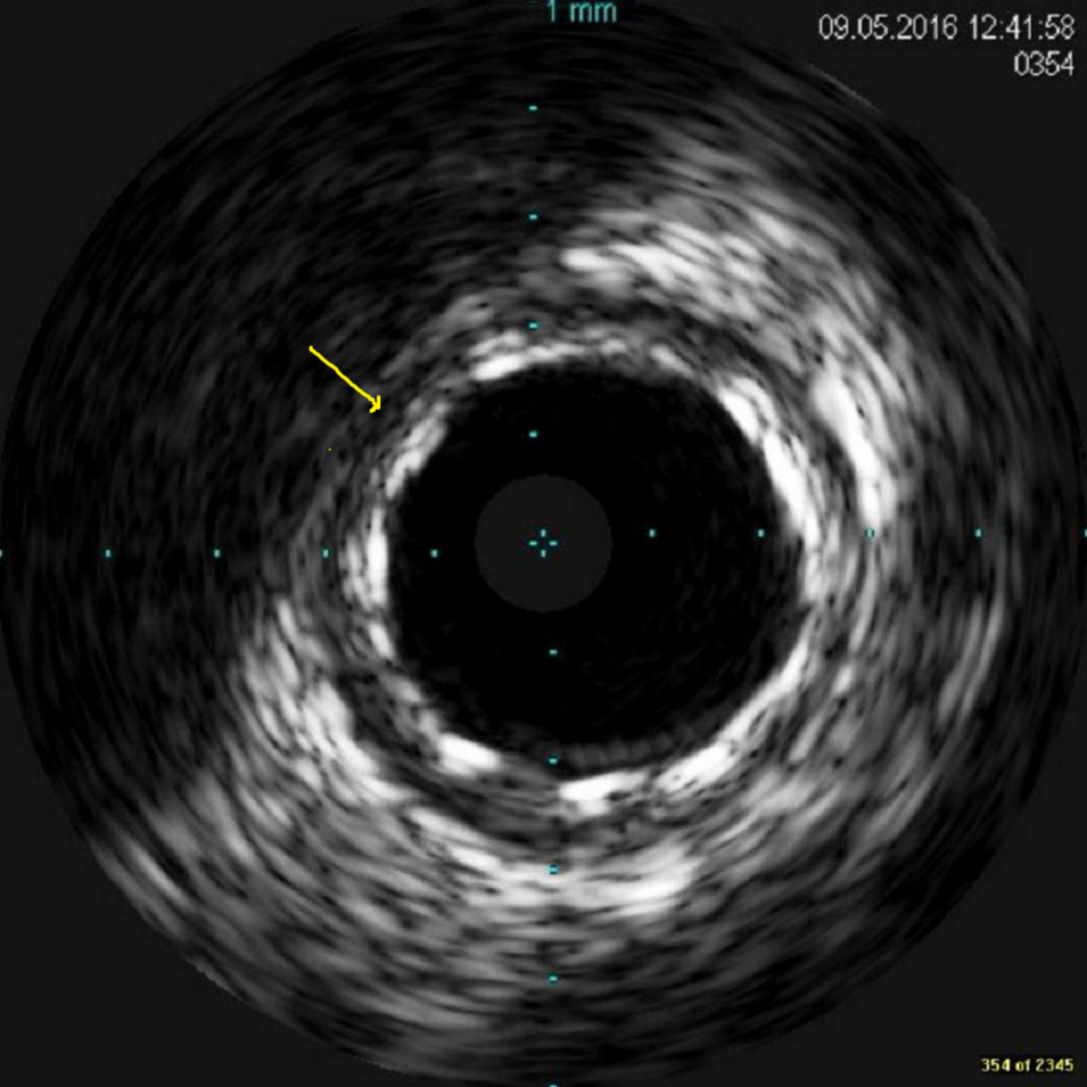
The final intravascular ultrasound image of the stented segment with excellent apposition
